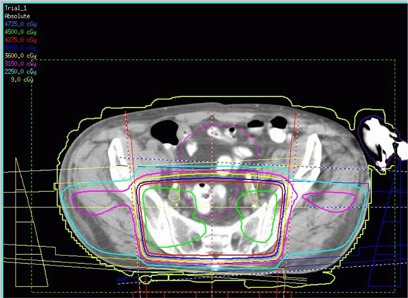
Axial view of the patient's CT‐based treatment plan. The LVAD pump is apparent on the right‐hand side of the figure. Three 15 MV photon beams are utilized, and 4500 cGy is prescribed in 25 fractions to the isocenter. The exit of the right lateral beam overlaps with the pump slightly. The isodose lines displayed are as follows: 4500 cGy (green), 4275 (red), 4050 (blue), 3600 (yellow), 3150 (pink), 2250 (teal), 9 (lime green).
